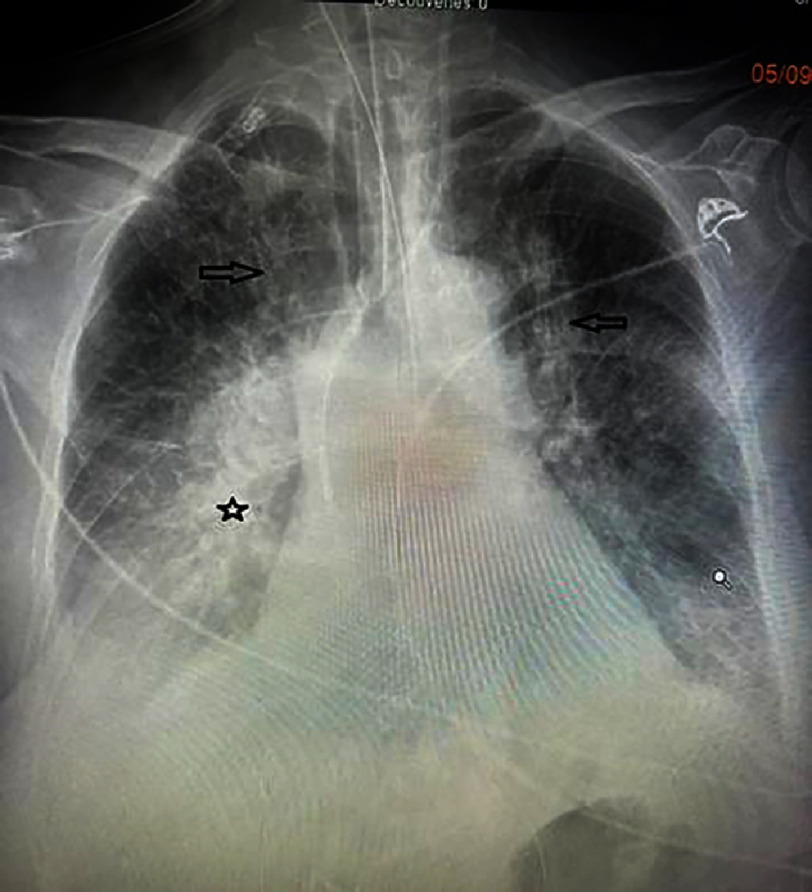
Chest X-ray (Patient #2) posteroanterior view showing an alveolo-interstitial syndrome (arrows) with a right basal focus (star).
